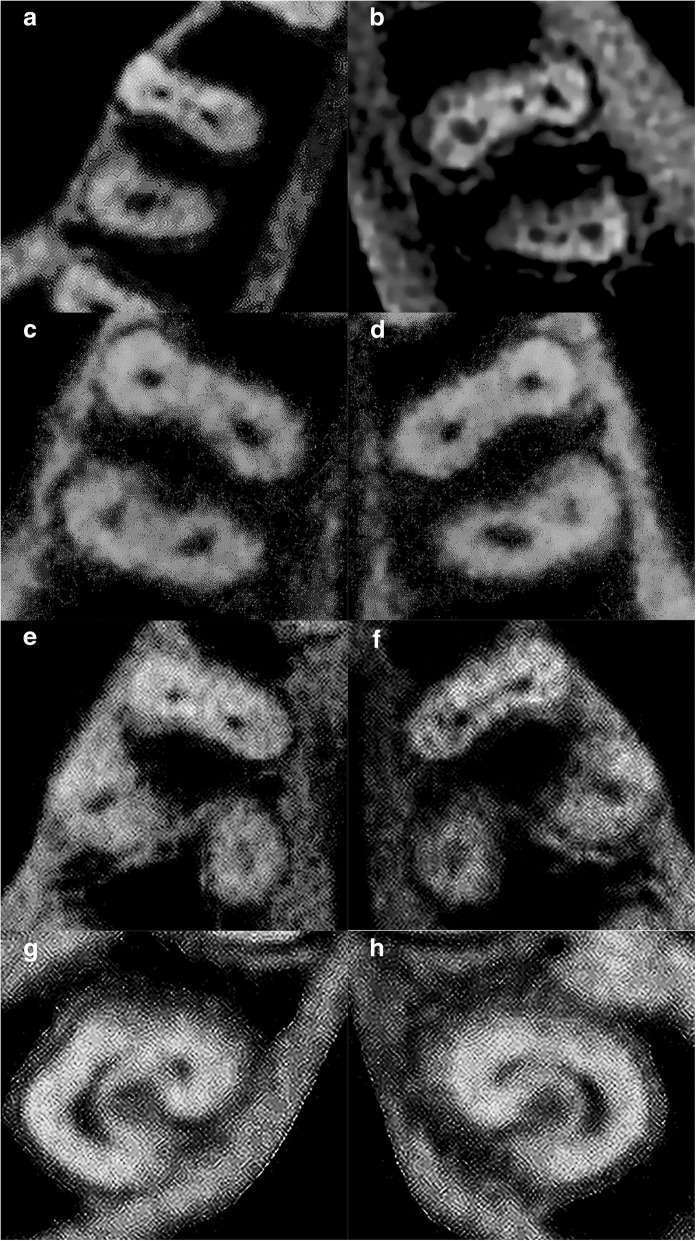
CBCT images of mandibular teeth. a Axial slice of a right mandibular first molar displaying a middle mesial canal in its mesial root. b Axial slice of a left mandibular first molar displaying a middle mesial canal in its mesial root. c Axial slice of a right mandibular first molar displaying a second distal canal in its distal root. d Axial slice of a left mandibular first molar displaying a second distal canal in its distal root. e Axial slice of a right mandibular first molar displaying an extra distolingual root (radix entomolaris). f Axial slice of a left mandibular first molar displaying an extra distolingual root (radix entomolaris). g Axial slice of a right mandibular second molar displaying a C-shaped canal. h Axial slice of a left mandibular second molar displaying a C-shaped canal
